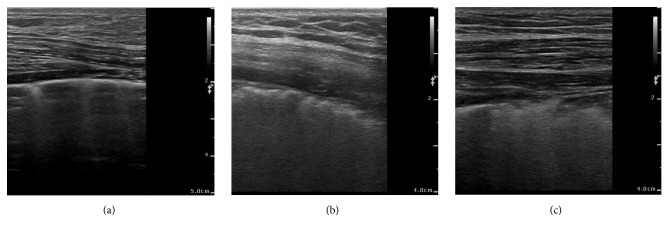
A linear probe was used to exclude noncardiac ULCs. (a) Normal pleura line and cardiac ULCs. (b, c) The abnormal pleural line could also generate ULCs (which are best visible under real-time examination), and they were confirmed by high-resolution computed tomography as interstitial lung disease and pneumonic infiltrate, respectively.
